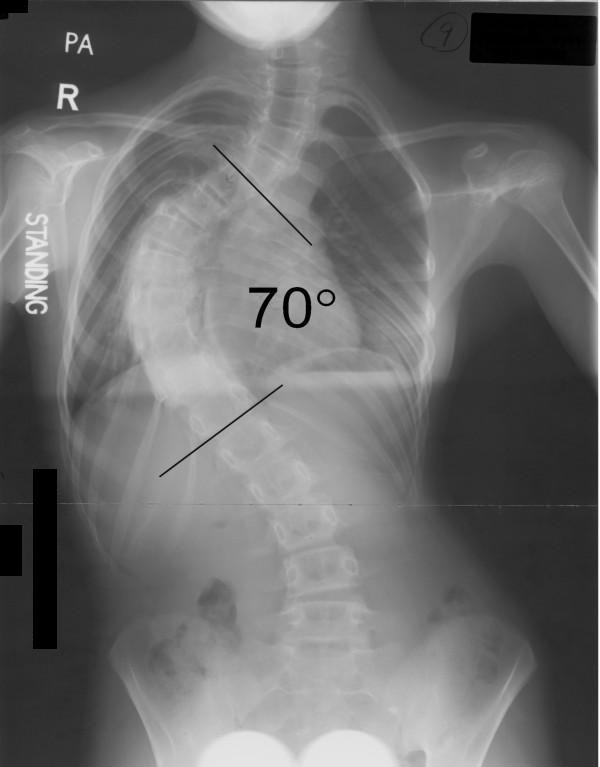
Standing radiograph before anterior release.
